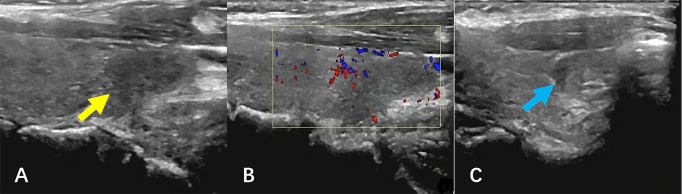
Ultrasound images after therapy. (A, B) The hypoechoic lesion was observed in the lower pole of the left lobe of the thyroid gland (yellow arrow), with an unclear boundary, a regular shape, and an uneven internal echo. CDFI revealed blood flow signals around the lesion. (C) A similar echo was seen in the middle pole of the right lobe of the thyroid gland (blue arrow), and CDFI showed no blood flow signal. CDFI, Color doppler flow imaging.
